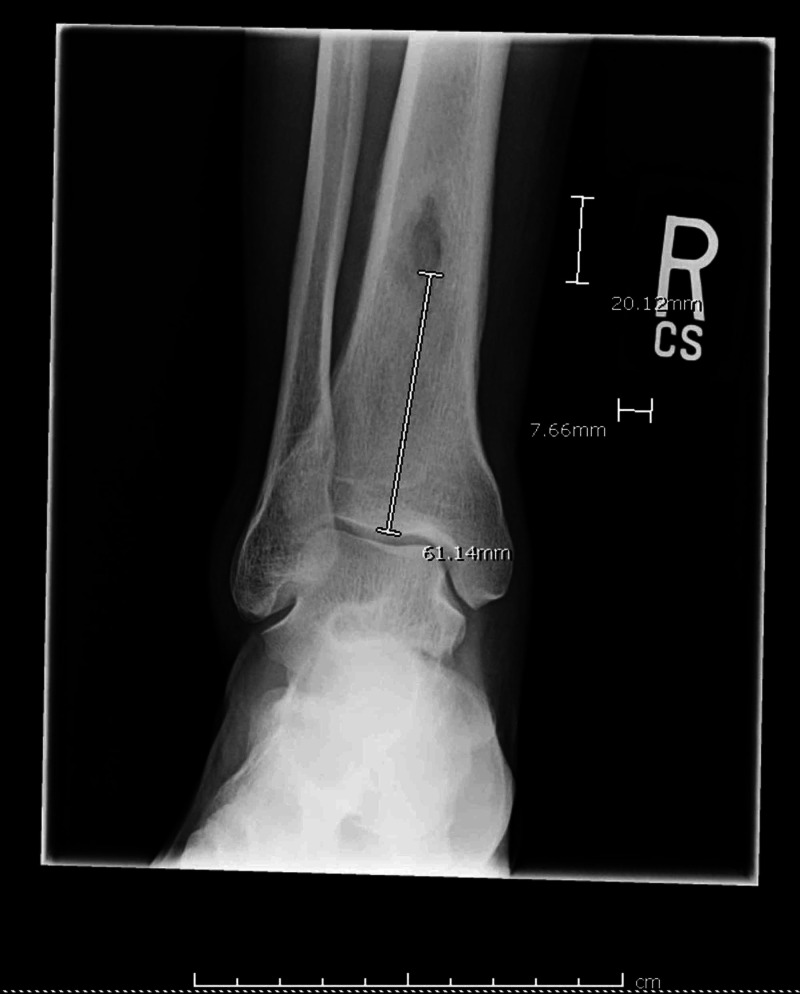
X-ray of the right ankle A 20-mm lesion seen in the distal tibia diaphysis was highly concerning for osteomyelitis with a Brodie's abscess given the aggressive periosteal reaction.
